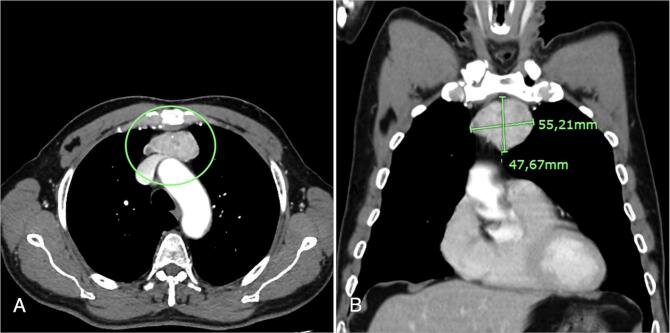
CT scan revealing a 55,21 × 47,67 mm tumour mass in the anterior and superior mediastinum (green circle); A – axial, B – coronal.
